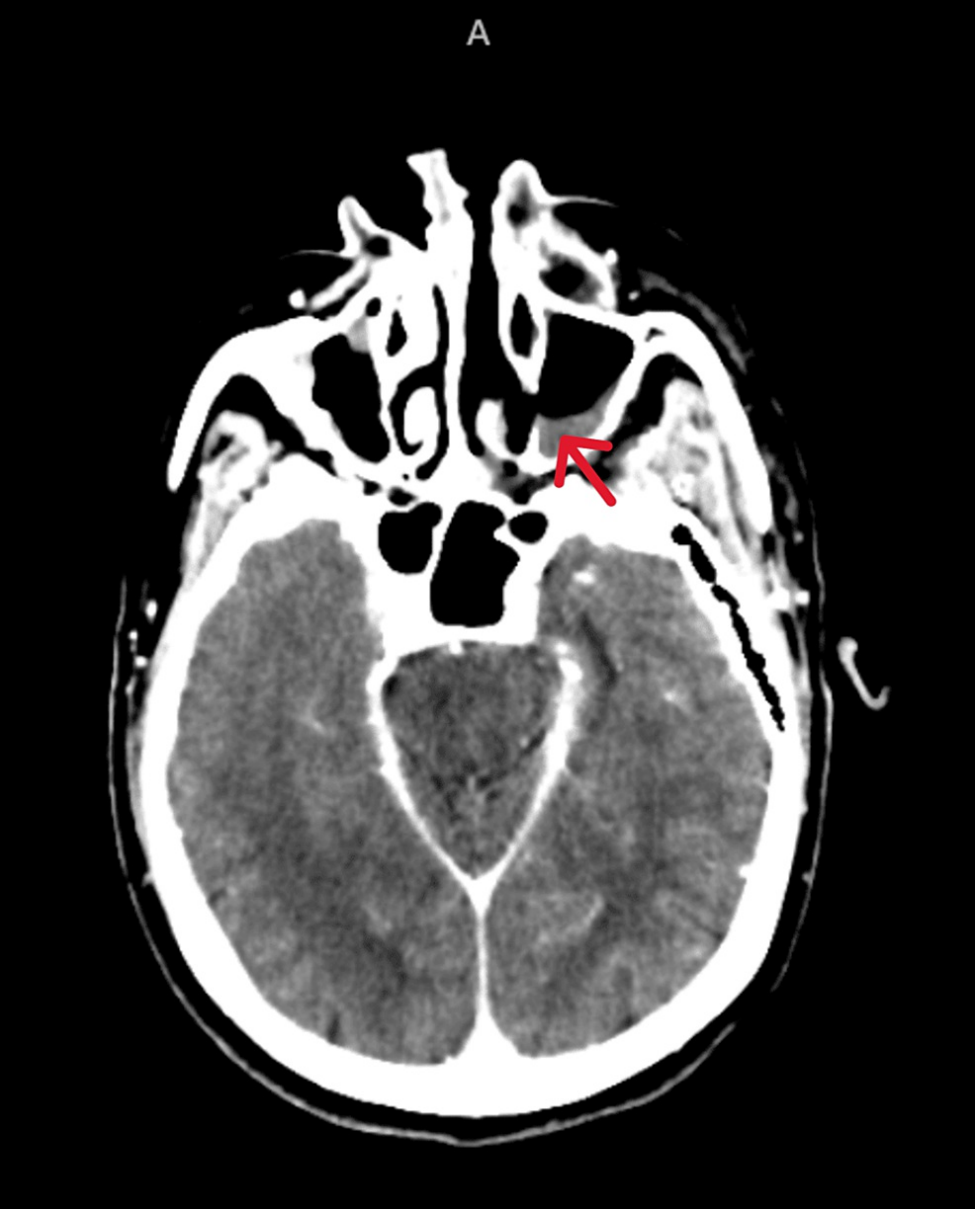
Contrast-enhanced computed tomography (CECT) brain and the orbits showing bilateral maxillary sinus opacification (Red arrow)
